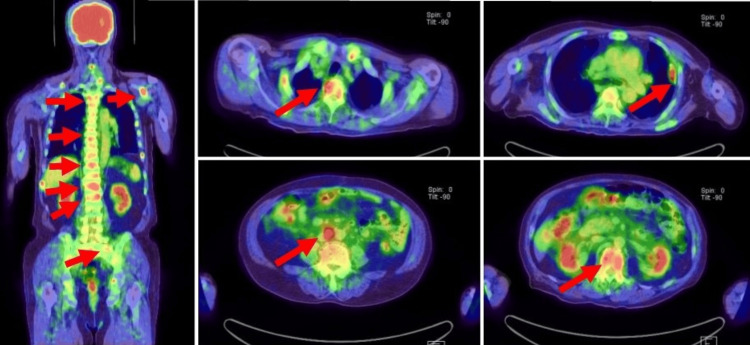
Evaluation of multiple metastases using 18F-fluorodeoxyglucose positron emission tomography-computed tomography. Six months after robot-assisted radical cystectomy, ^18^F-fluorodeoxyglucose positron emission tomography-computed tomography showed multiple bone metastases, para-aortic lymph node metastases, and pleural metastases (red arrowheads).
